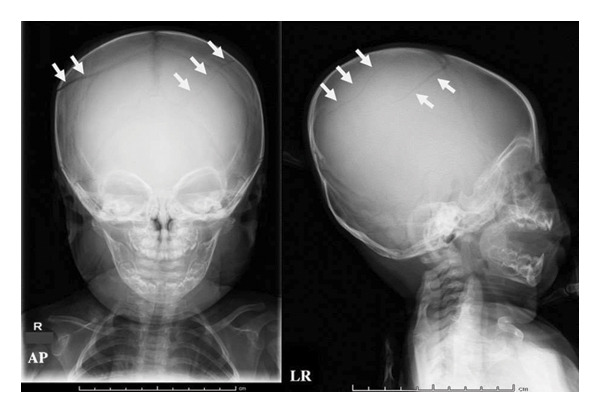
Skull radiography shows bilateral linear skull fractures (white arrows).
